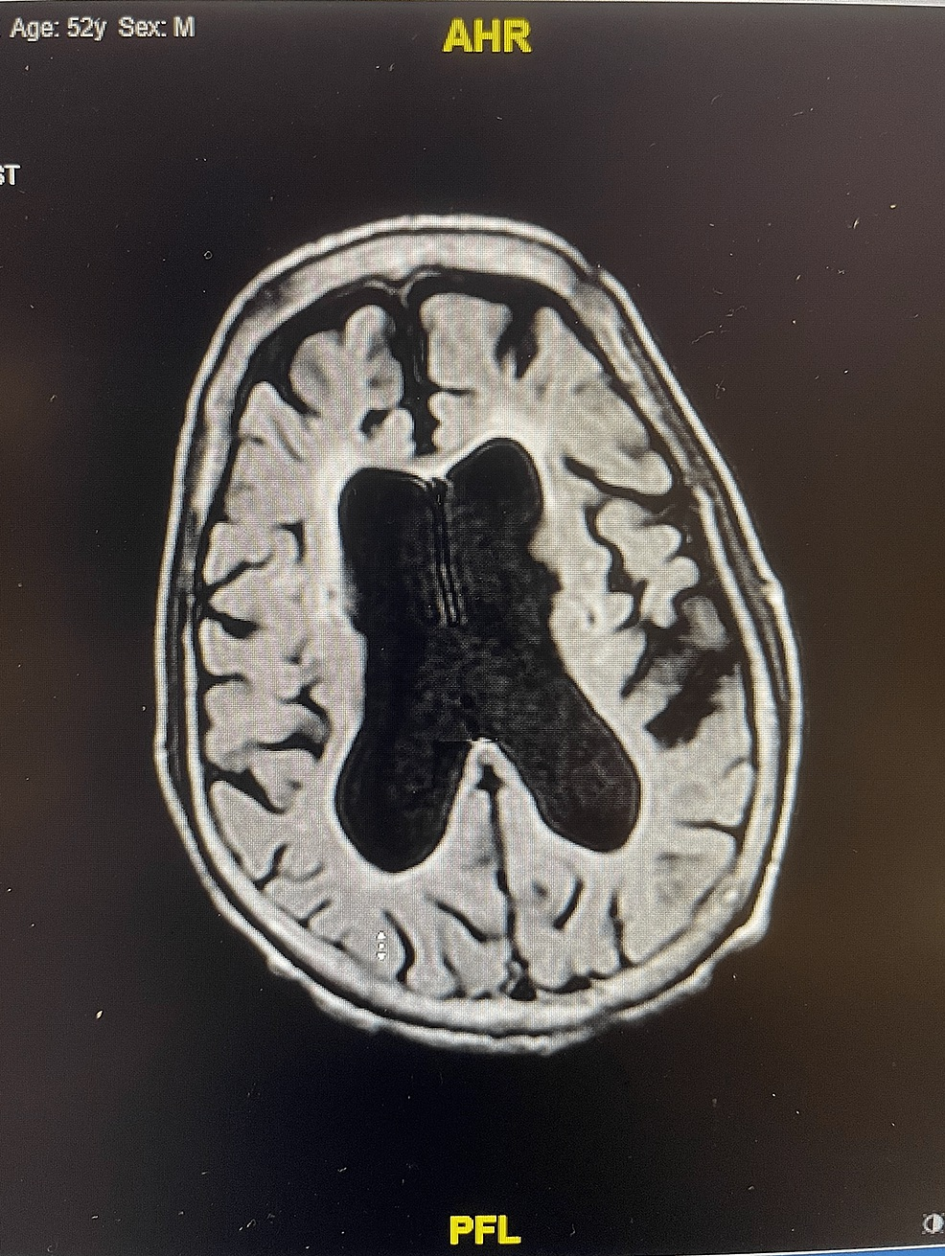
Brain MRI showing enlarged ventricles with moderately prominent cortical atrophy and no acute abnormalities. MRI, magnetic resonance imaging
